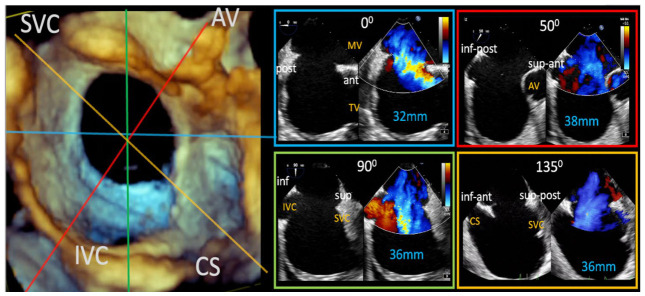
TOE protocol for ASD assessment. 3D echo image (3D live zoom mode) en faces right atrial (RA) view with landmarks. Left panel depicts the four standardized views. Each coloured box relates to each coloured line 0, 50, 90, 135 degrees, which demonstrate the key anatomical landmarks in each view. The measurements of the ASD are shown, colour Doppler is applied and the largest diameter of the defect is found (typically around end ventricular systole) in the cardiac cycle and the measurement is made from edge to edge of the colour flow image. The measurements are then averaged. For device sizing see Table 2 and Figure 3. The ASDs depicted have minimal rims for much of its circumference and was deemed unsuitable for transcatheter device closure. *SVC superior vena cava, AV aortic valve, CS coronary sinus, IVC inferior vena cava, MV mitral valve, tricuspid valve*.
